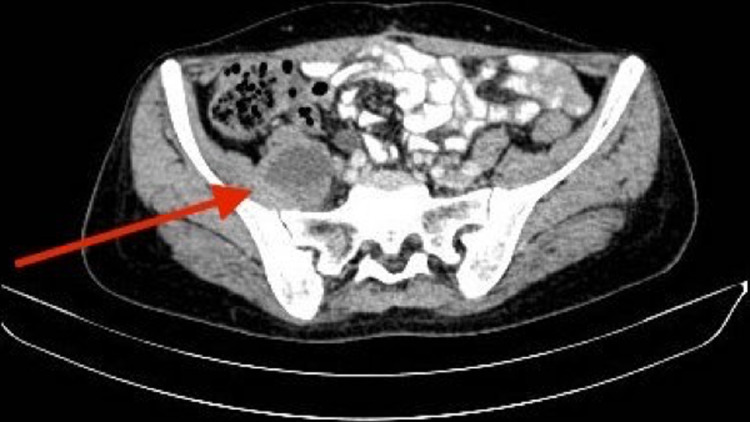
computed tomography showing a well-defined hypodense lesion in the right psoas muscle, measuring 4 x 3.6 x 3 cm
